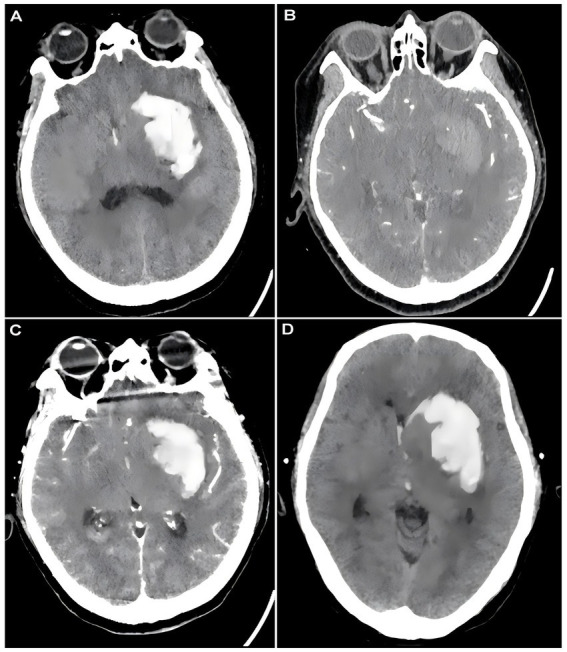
A 37-year-old woman with hemorrhage in the left basal ganglia, external capsule, insula, paraventricular, and frontal lobes. The onset time was 7:26 on 17 January, and the time of arrival at the emergency department of our hospital was 10:36. (A,B) First CT and enhanced CT at 10:55, hematoma volume was 27.48 mL, spot sign was negative; (C) sequential CT scan at 10:56 with brush positive and isolated morphology; and (D) re-examined CT at 14:59, the hematoma volume was 29.07 mL without significant hematoma expansion.
